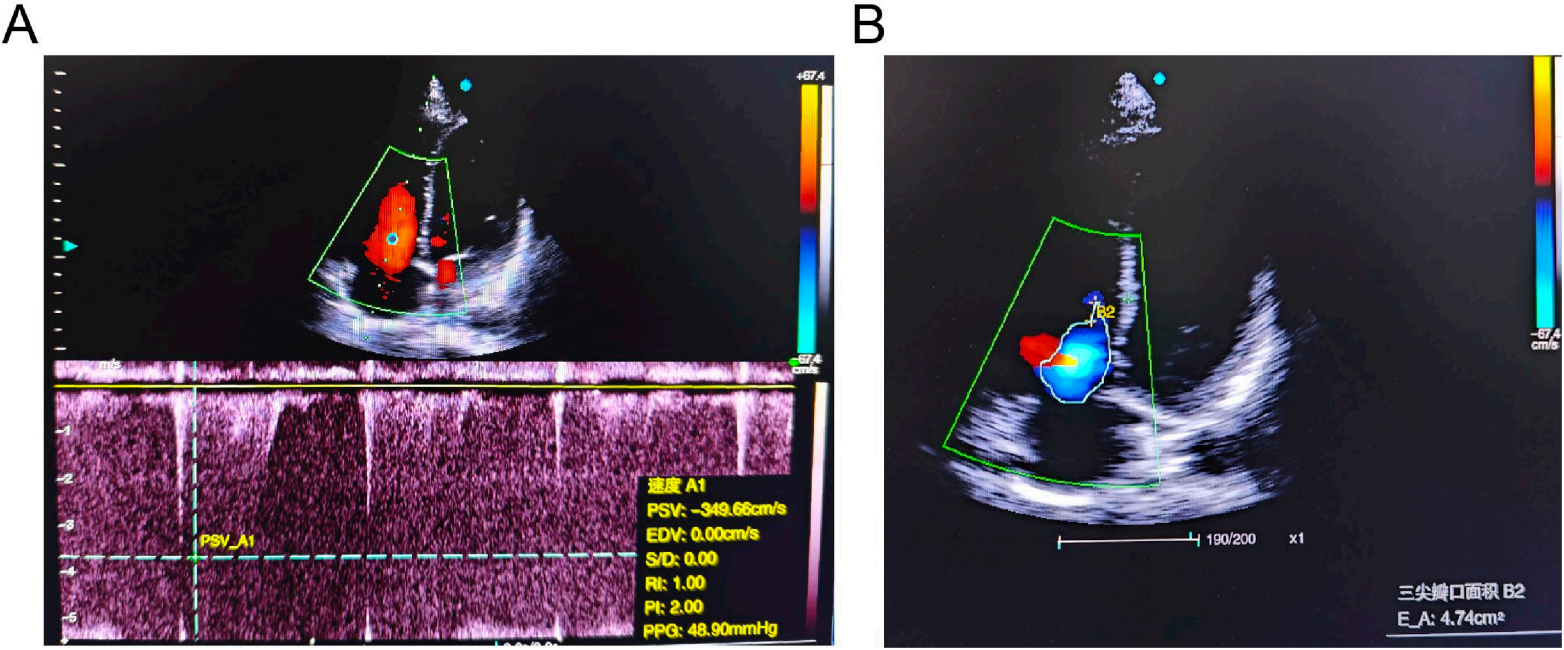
Echocardiographic findings of the patient. (A) Continuous-wave Doppler spectrum showing tricuspid regurgitation. (B) Apical four-chamber view demonstrating significant tricuspid regurgitation (TR).
